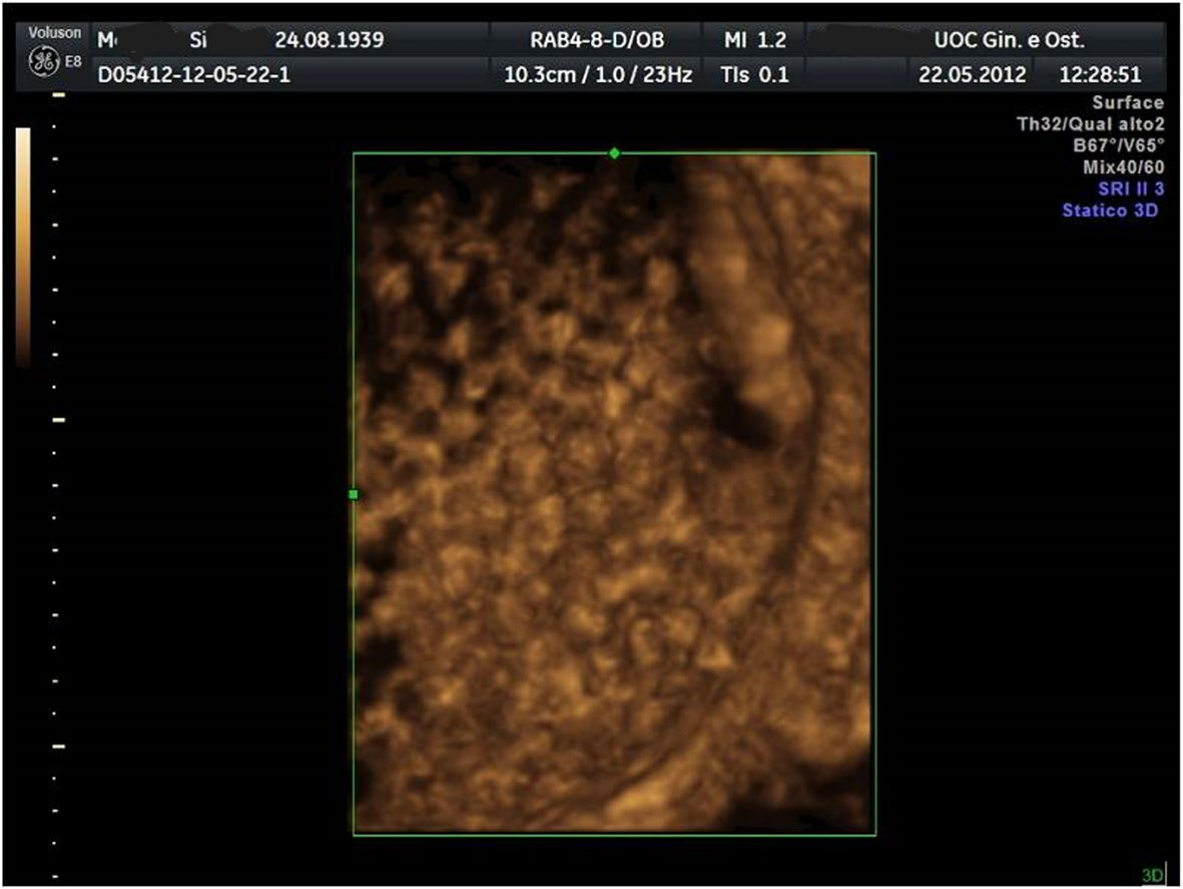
Static tridimensional (3D) ultrasound showing the “honeycomb” aspect
